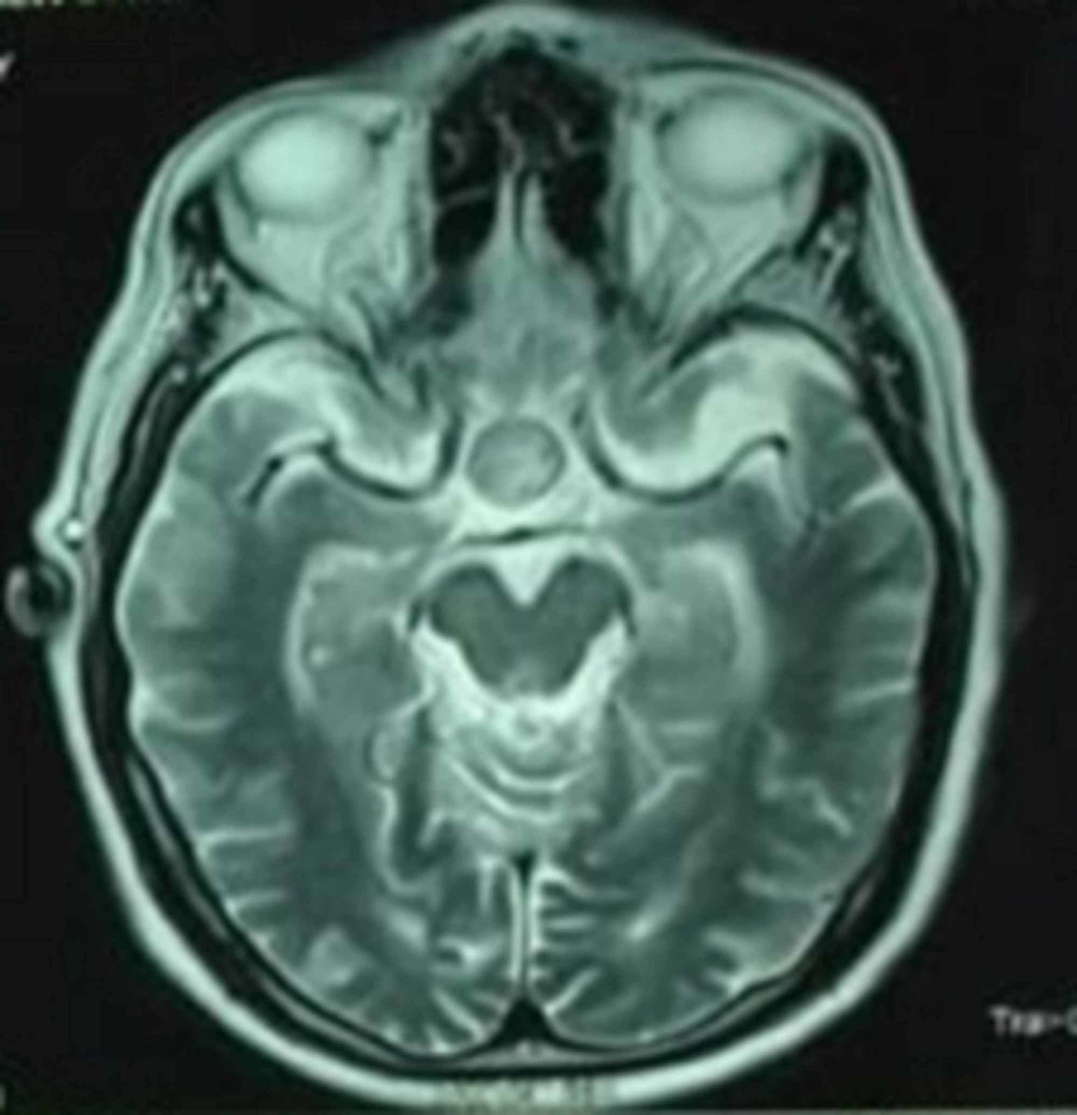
MRI brain showing pituitary macroadenoma MRI: magnetic resonance imaging
